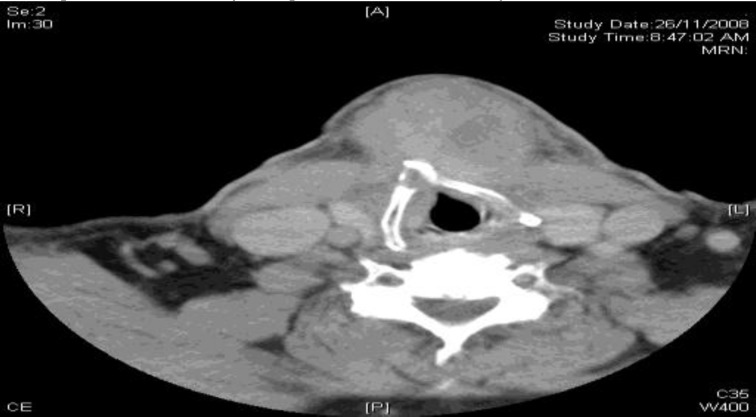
Axial CT scan of the neck at the level of hyoid bone demonstrating ill-defined low attenuation rounded lesions with thick wall in the anterior neck
